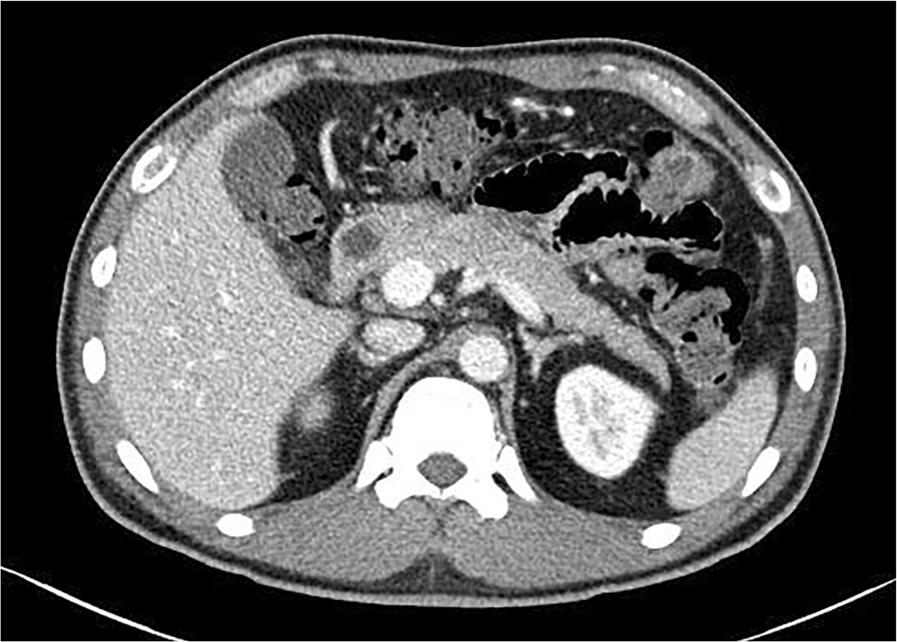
Computed tomography of pancreas. There was no evidence of pancreas mass or pancreatitis
